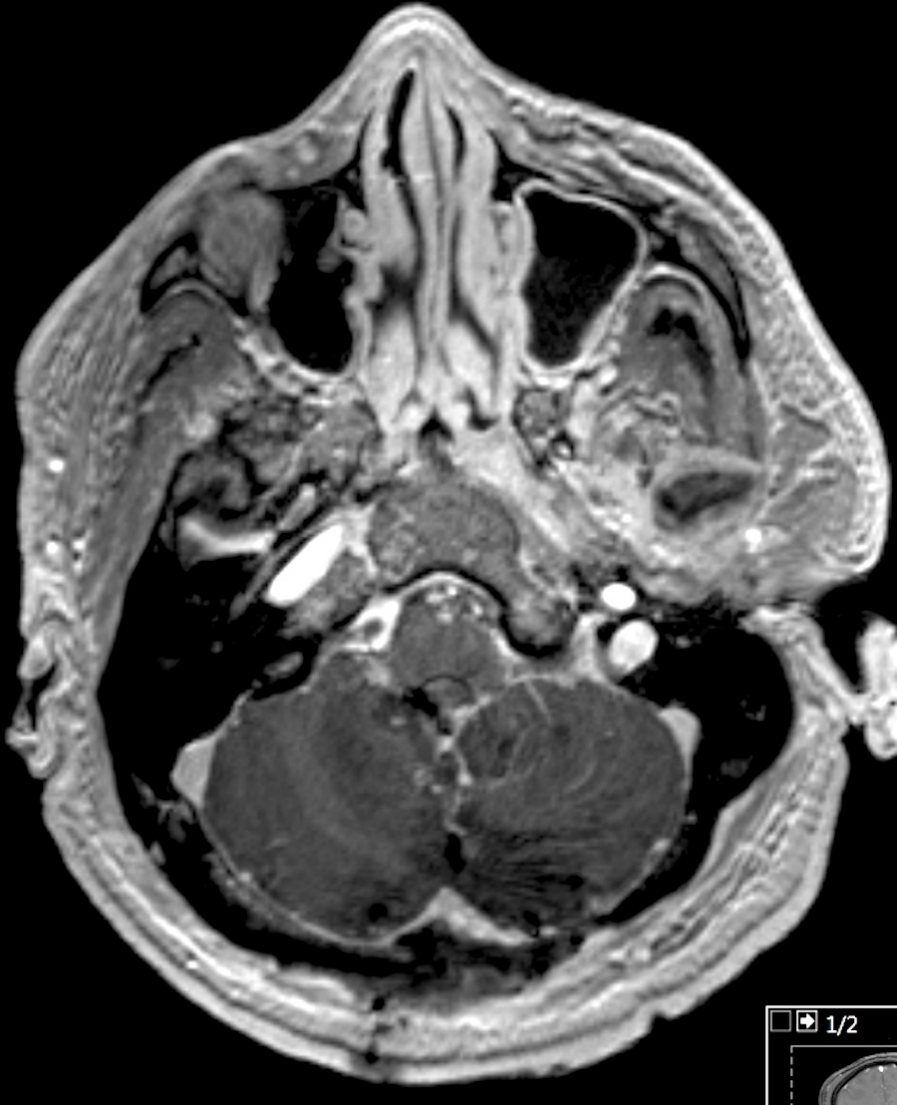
Postoperative magnetic resonance imaging (MRI). Post-gadolinium postoperative MRI demonstrating extent of resection and operative changes.
